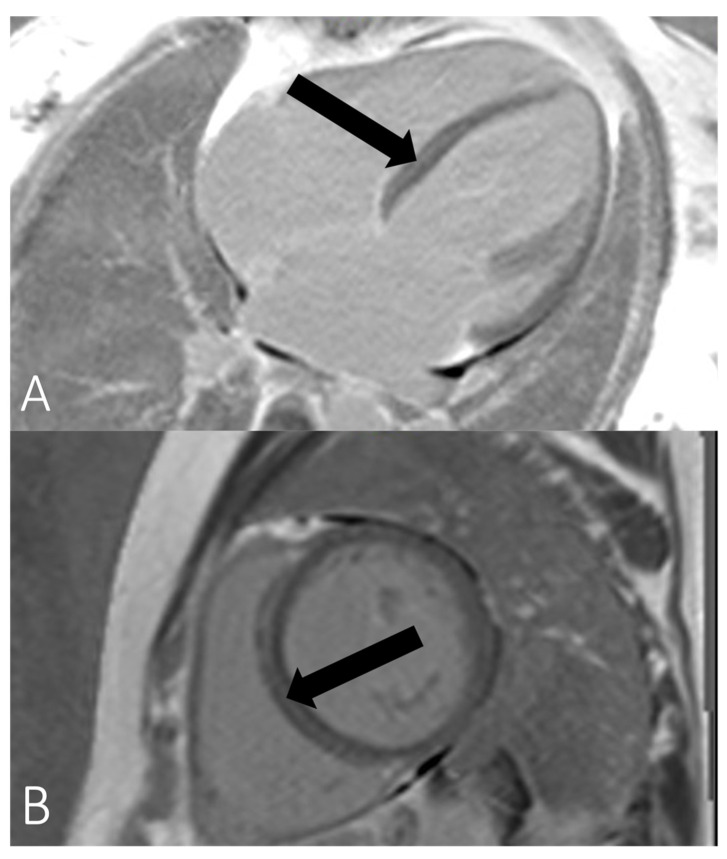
Magnetic resonance imaging (MRI) samples of patient III:3. Septal midwall late-gadolinium enhancement (LGE) in (A) long-axis view and (B) short axis view, indicated by black arrows.
